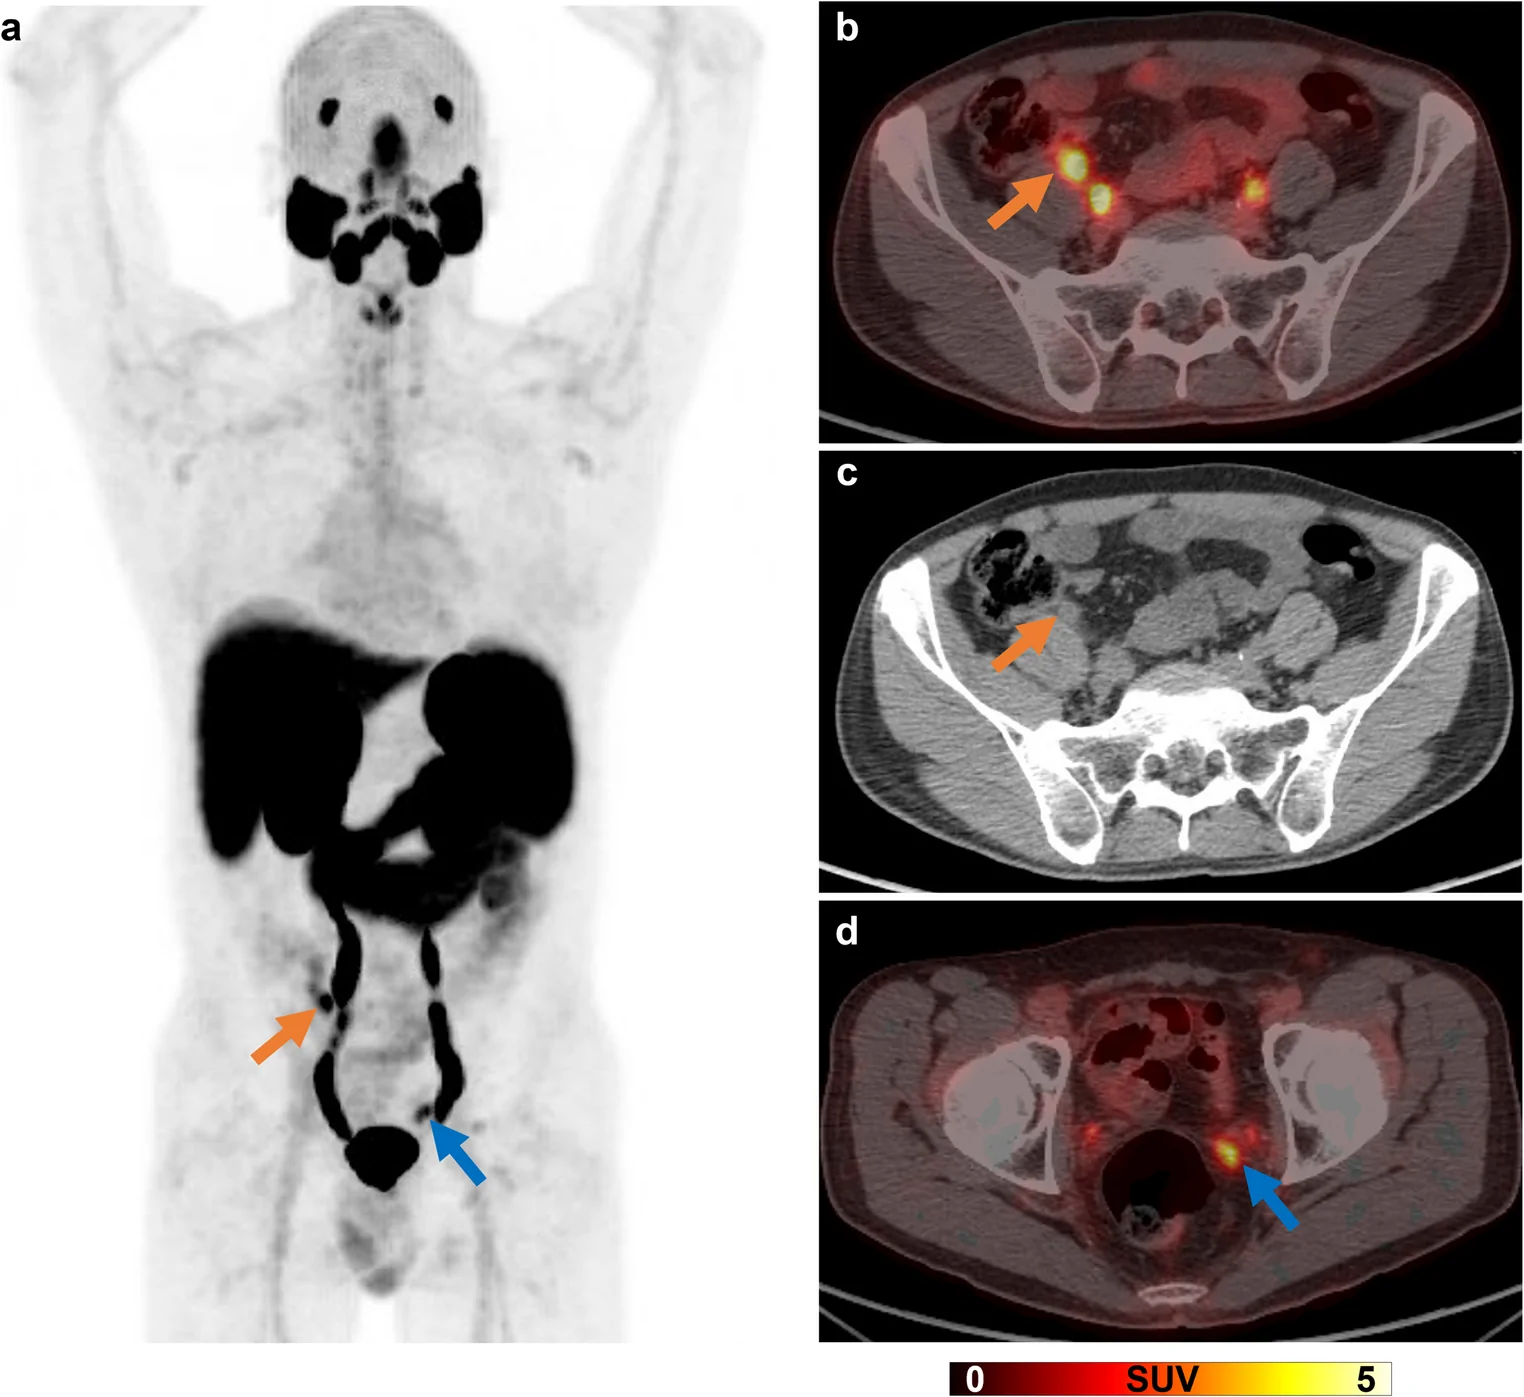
Patient with PSMA-avid appendiceal metastasis and left seminal vesicle bed recurrence. 56-year-old man with prostate cancer (Gleason 4+3) post-prostatectomy, ADT, and salvage RT, with rising PSA (0.8 ng/mL). (a) Maximum-intensity-projection (MIP) image showed focal uptake in the right lower quadrant (orange arrow). (b) Axial fused PET/CT and (c) axial CT image showed the uptake localized to the thickened appendix. Morphological appearance suggested appendicitis, and subsequent hemicolectomy confirmed appendiceal metastasis from prostate cancer. Uptake in left seminal vesicle bed (blue arrow) in (a) MIP and (d) fused PET/CT image, suspicious for tumor recurrence
